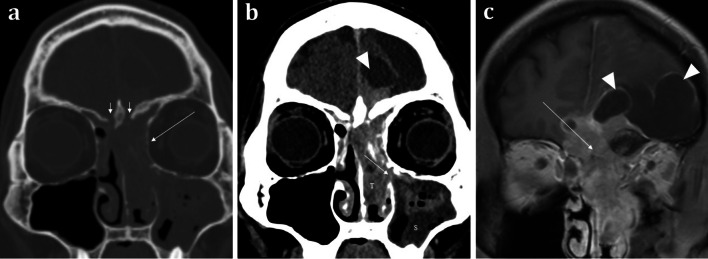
Olfactory neuroblastoma (Esthesioneuroblastoma) in a 41-year-old male presenting with a 2-month history of change in behaviour and anosmia. Coronal bone (a) and soft tissue window (b) CT images demonstrate a large anterior skull base mass centred upon the olfactory neuroepithelium. There is extensive bone destruction of the left and right cribriform plates and turbinates (short arrows, a), and thinning of the medial wall of the left orbit (long arrow, a). There is also inferior extension of the tumour (T) through the ethmoid air cells into the nasal cavity and extending through the left middle meatus into the maxillary sinus (arrowed, b). The soft tissue density of the tumour (T) is distinct from the left maxillary sinus fluid contents (S). Note the fluid density intracranial “capping cysts” at the left frontal lobe (arrowhead, b). Coronal T1WI with contrast (c) depicts a tumour with moderately intense contrast uptake, conforming to a “dumbbell-shape” with a “waist” at the cribriform plate (arrowed, c). Again, note the presence of T1 hypointense “capping cysts” at the intracranial margin, which are a distinguishing feature of these tumours (arrowheads, c)
